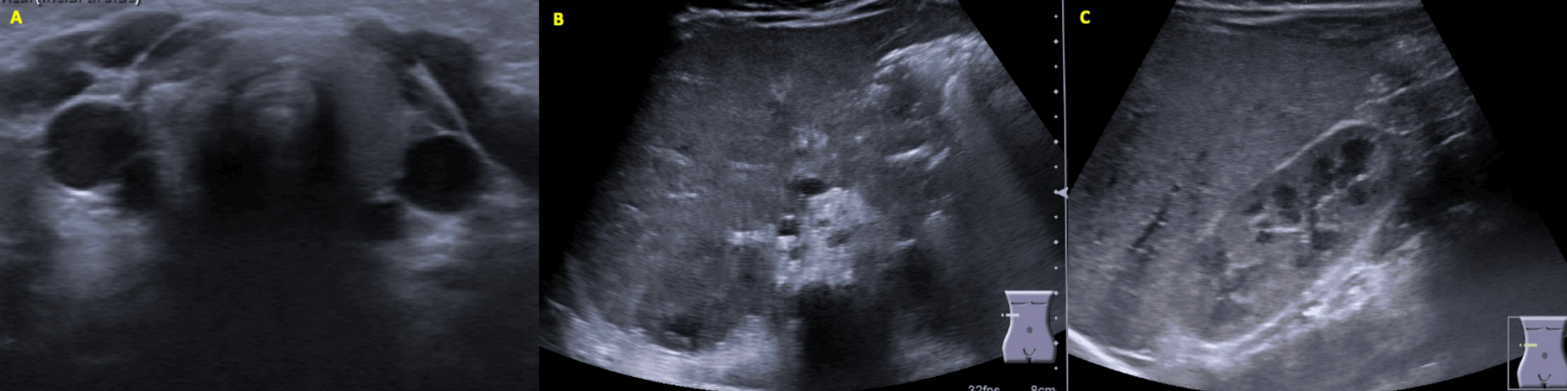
Serial images of thyroid (A), hepatic (B), and renal (C) ultrasound.
